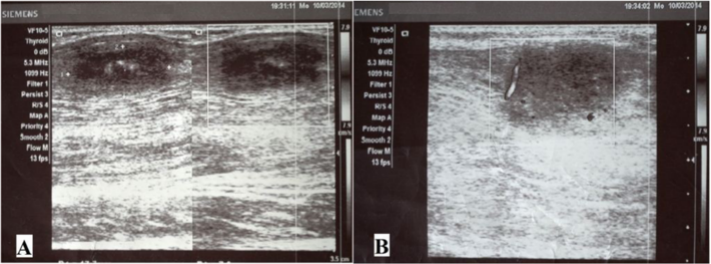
Ultrasonography of swellings. A. Well-defined hypoechoic nodules measuring 1.7cm×2.4cm were seen in the calf muscle. There were associated echogenic foci seen in the centre of the lesion suggesting calcification. B. On colour Doppler minimal peripheral vascularity was noted.
